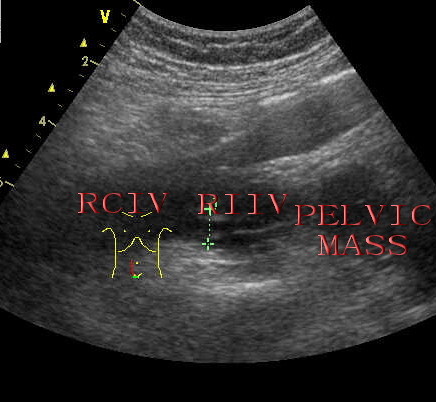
Pelvic view showing a pelvic mass and continued medium echogenic oval tumor mass within right internal iliac vein, right common iliac vein by abdominal ultrasound. RIIV: right internal iliac vein, RCIV: right common iliac vein.
